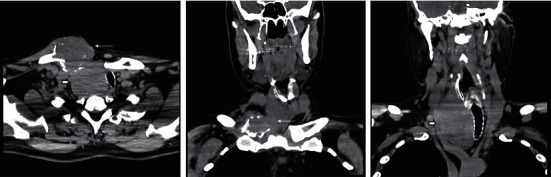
Axial CT image of the soft tissues of the neck (left image) demonstrating a heterogeneous mass within the right clavicular head, which is expansile with associated bony destruction (thin white arrow). There is also mass-like enlargement of the right thyroid lobe (short thick arrow). A coronal CT image of the soft tissues of the neck (middle image) also shows the destructive mass in the right clavicular head with soft tissue component (thin white arrow). A coronal CT image of the neck soft tissues more posterior in position (right image) demonstrates the mass-like enlargement of the right thyroid lobe (short thick arrow).
